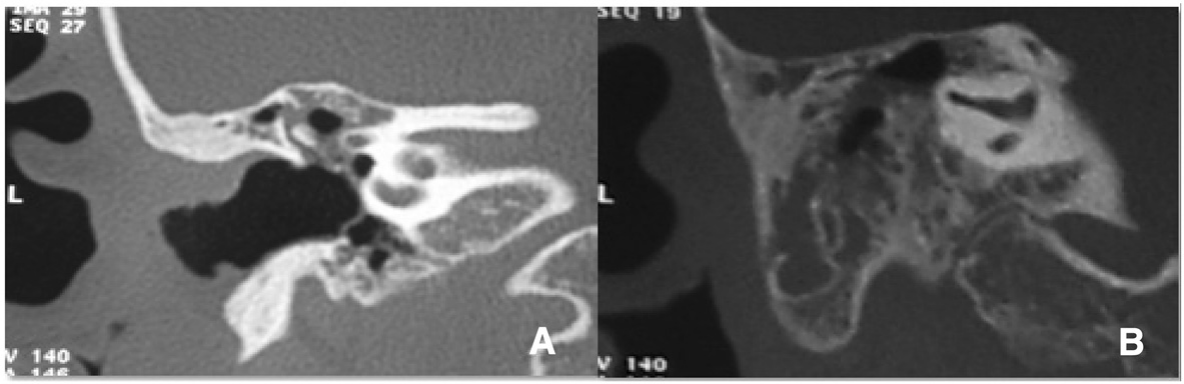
Computed tomographic scans taken before the tympanoplasty. a Coronal computed tomographic scan showing an occupying lesion in the attic compatible with cholesteatoma. b No abnormality in the left temporal bone ossification was found
